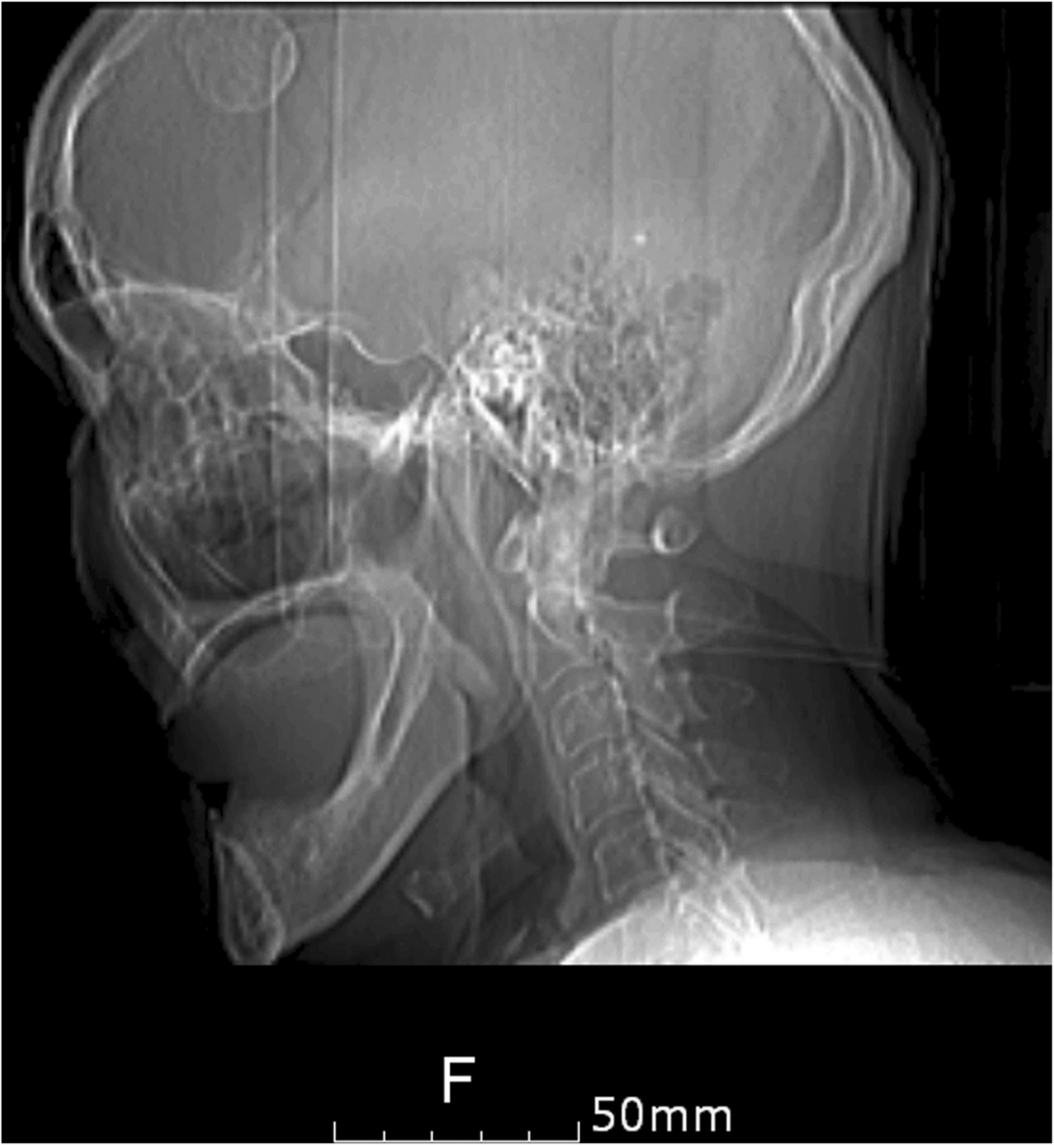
Scout film of the petrous bone computed tomography (CT) scan. It showed a dense radiopaque mass in the frontal area.
